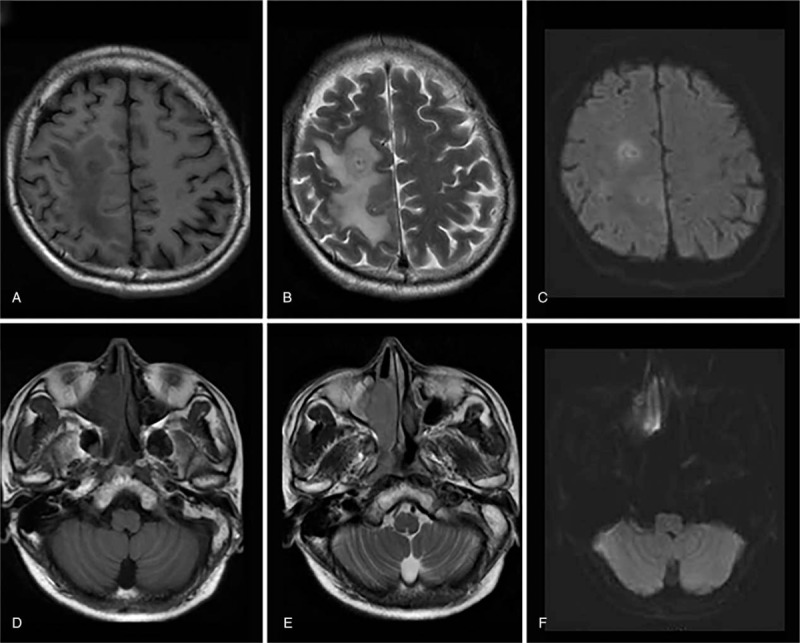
Repeated MRI. A–C, Cranial involvement increased in size. D, E, An isointense mass (arrow) in the region of right maxillary sinus. F, Axial DWI shows restricted diffusion of the lesion.
